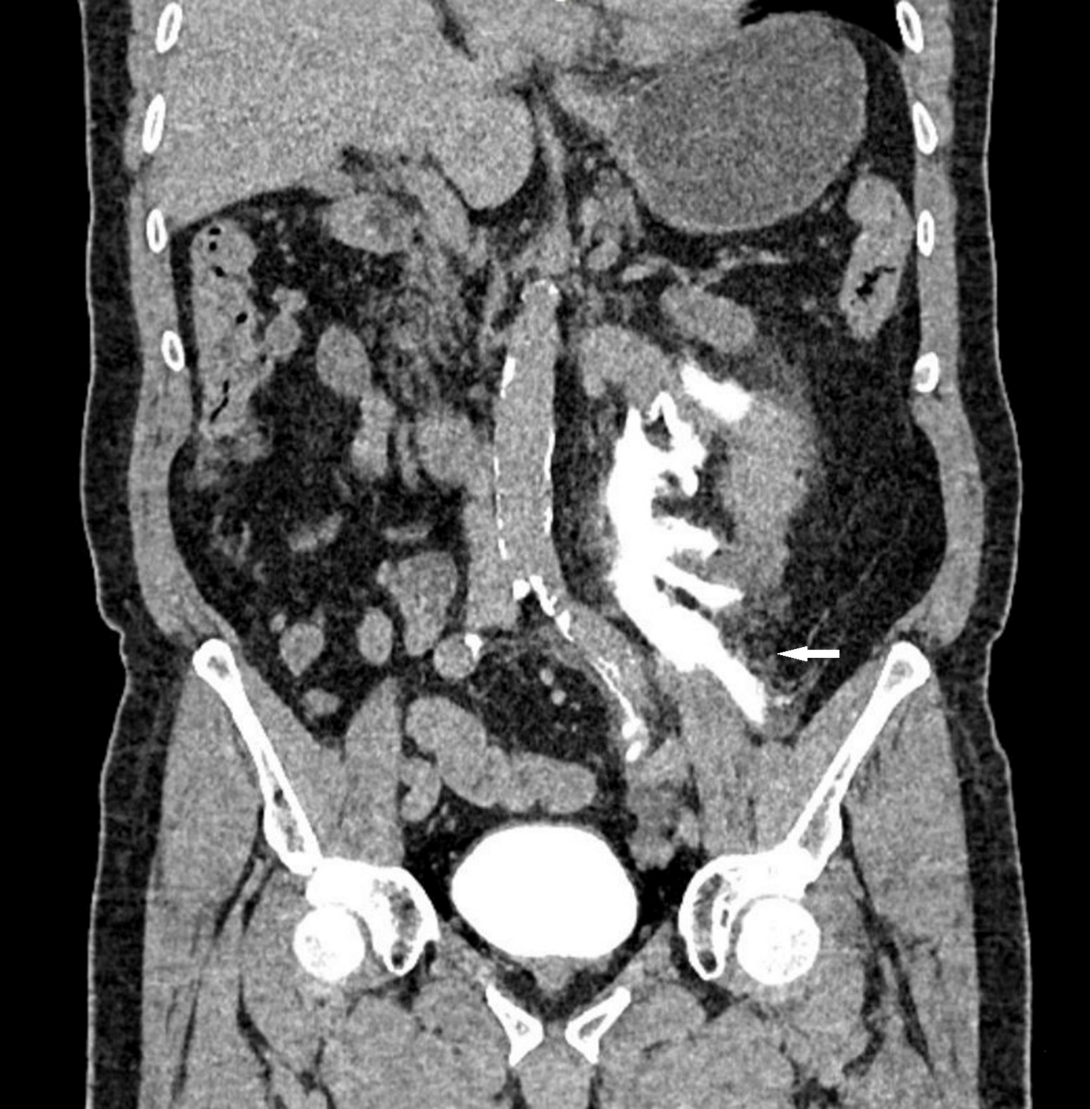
Coronal CT image obtained after intravenous contrast administration demonstrating leakage of contrast material from the proximal left ureter, consistent with ureteral rupture.
